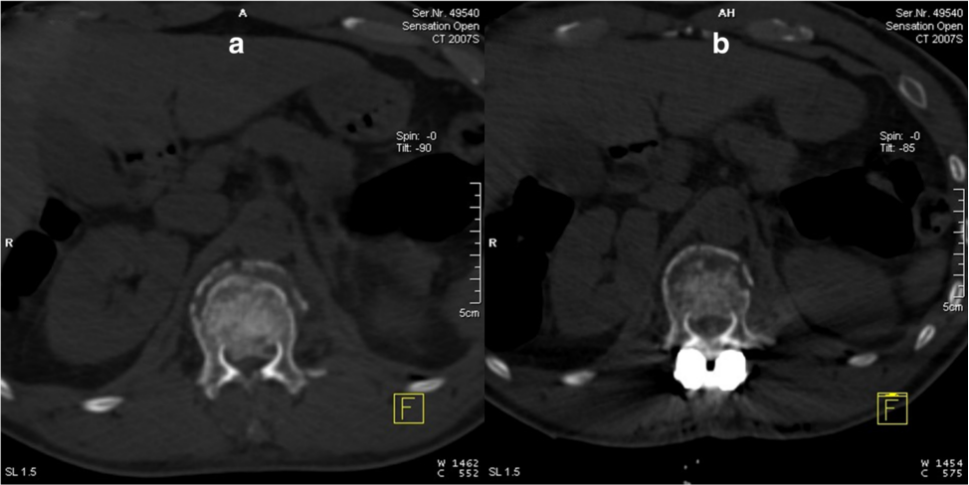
a CT picture of intra-canal fracture fragment before PLLR. b CT picture of intra-canal fracture fragment after PLLR
